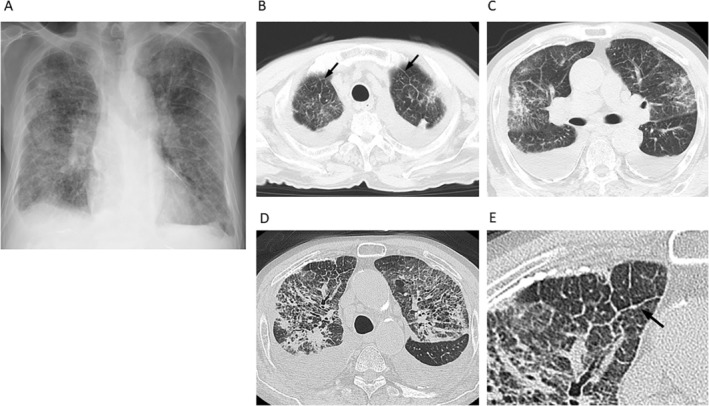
Chest X‐ray and computed tomography (CT) on admission. (A) Chest X‐ray showed bilateral ground‐grass opacity, consolidation, and blunting of the bilateral costophrenic sulci. (B, C) Chest CT revealed bilateral ground‐grass opacity and partial consolidation with upper lobe predominance. Smooth thickened interlobular septa were seen only in the bilateral lung apexes (arrows). (D, E) High‐resolution CT on hospital day 7. The thickened interlobular septa were slightly irregular (arrow).
